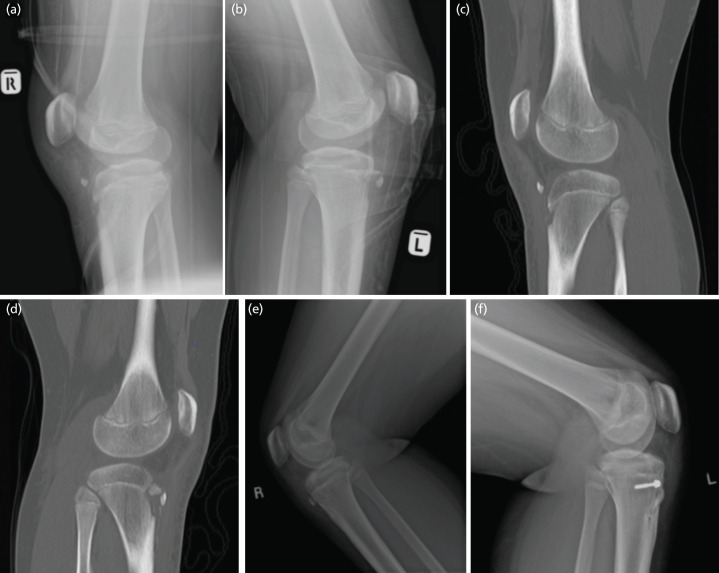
(a, b) Lateral view plain radiograph of right and left knee showing the displacement of the tibial tuberosity avulsion fracture. (c) CT sagittal view of right knee showing avulsed fragment at right knee measuring 0.5 x 1.4 x 0.8cm with approximately 2.4cm displacement from the tibial tuberosity. (d) CT sagittal view of left knee showing two fragments were seen at left knee measuring 1.0 x 0.4 x 1.5cm and 1.1 x 2.1 x 1.5cm, respectively, which were 1.2cm displaced. No intraarticular extension seen on both knees. (e, f) Lateral view plain radiograph at six months post operation showing reduced tibial tuberosity fragment of bilateral knees with in-situ screw at left tibial tuberosity.
